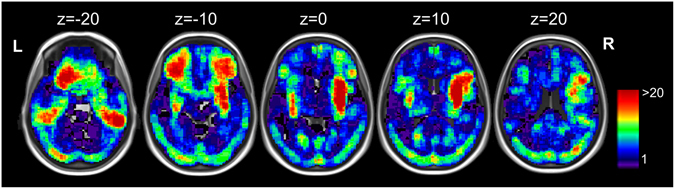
Lesion overlap of all patients (*n* = 153) presented on axial slices from caudal (z = −20) to cranial (z = 20) from a standard MRI template. Scale bars represent t-scores. L: left hemisphere, R: right hemisphere. Note that z-coordinates relate to MNI space.
